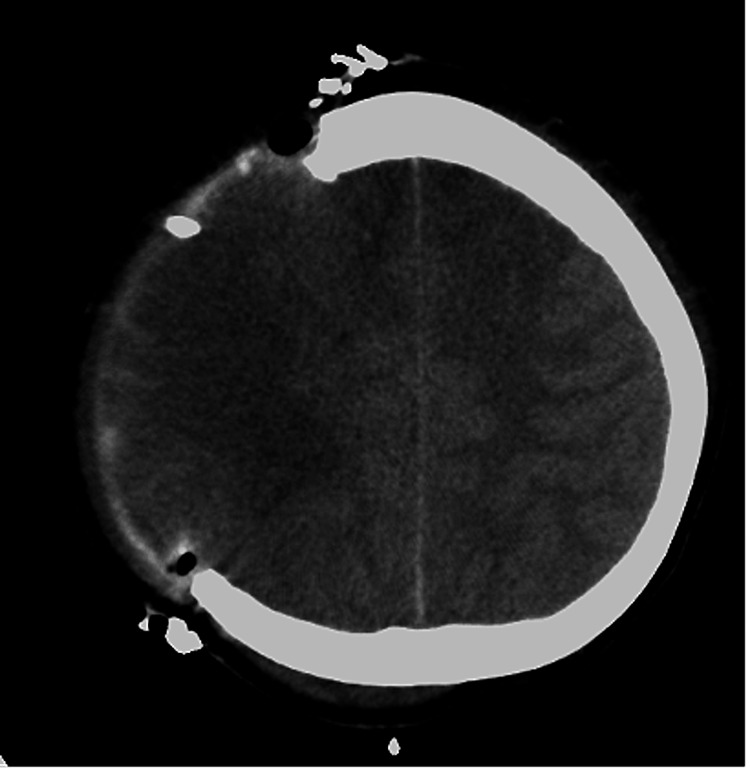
A CT scan of the head on the eighth day from onset showed an ischemic lesion in the left anterior cerebral artery territory. CT: computed tomography
